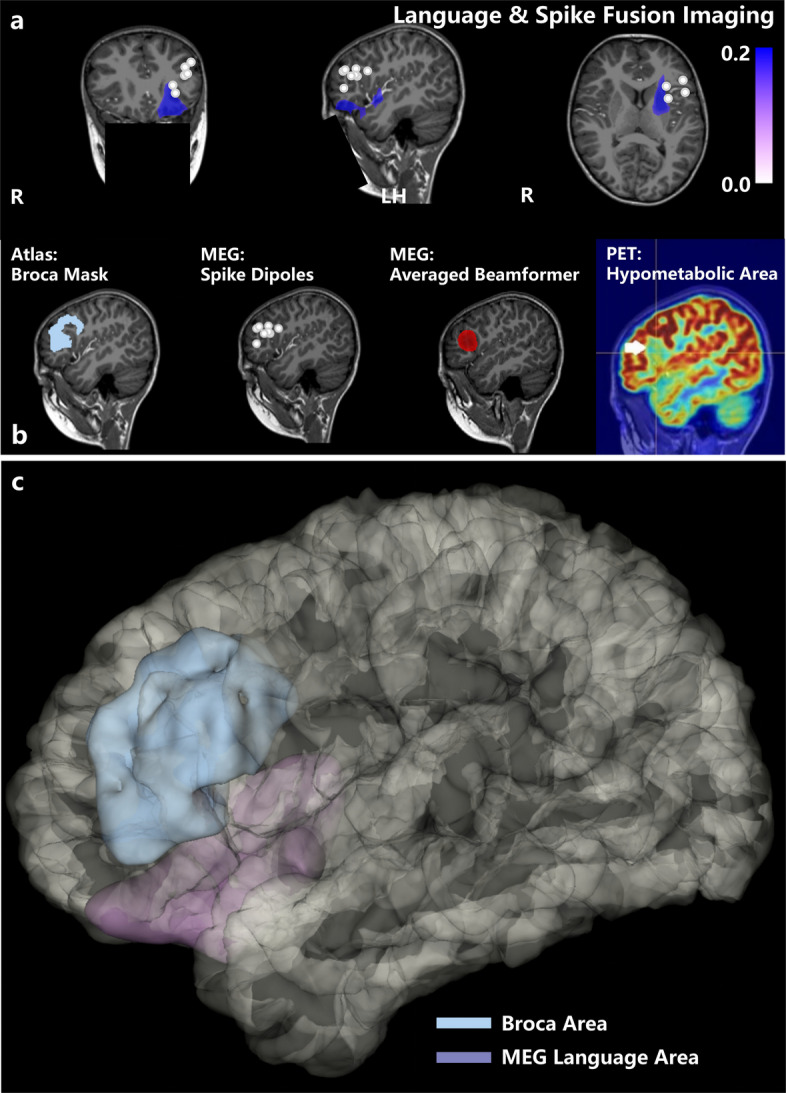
a overlays the dipole localizations with the language beamformer results, where the language paradigm localization demonstrates a predominant left-hemisphere dominance and high-signal areas include the left orbitofrontal cortex, insula and superior temporal gyrus. The overlap between the two datasets is minimal and the language distribution significantly differs from the classical Broca's area. b shows the sagittal slice of the subject's left hemisphere language area. From left to right, the first column represents the Brodmann area Broca region mask, the second column displays the epileptic discharge region localized by MEG, the third column displays the MEG spikes averaged beamformer results and the fourth column shows the hypometabolic region identified by PET-CT. The spatial locations of these four regions are highly consistent. c presents the differences between the subject's Broca area and the MEG-based language localization in the surface space. The MEG threshold is set to 60% of the maximum, and no overlap is observed between the two regions
